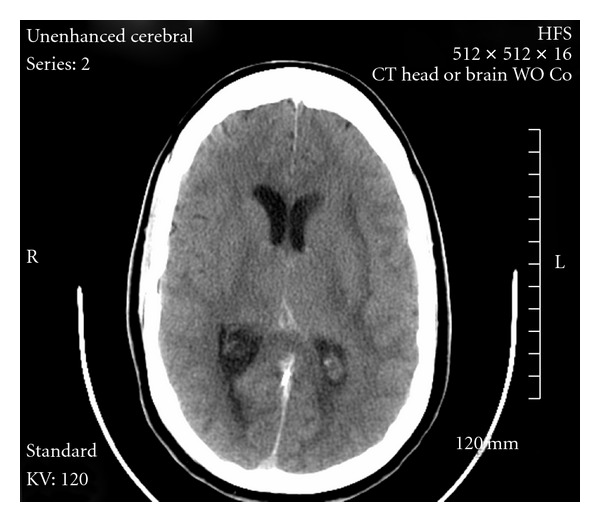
Noncontrast CT scan 1 hour after intrasinus rt-PA.
